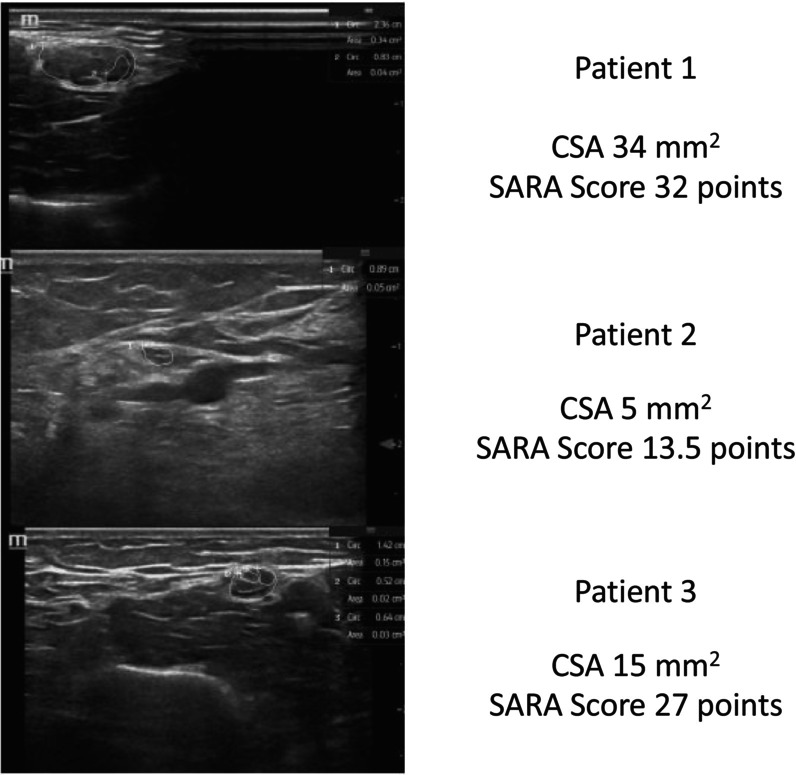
Comparison of high‐resolution ultrasound of the median nerve in the upper arm between 3 patients.
